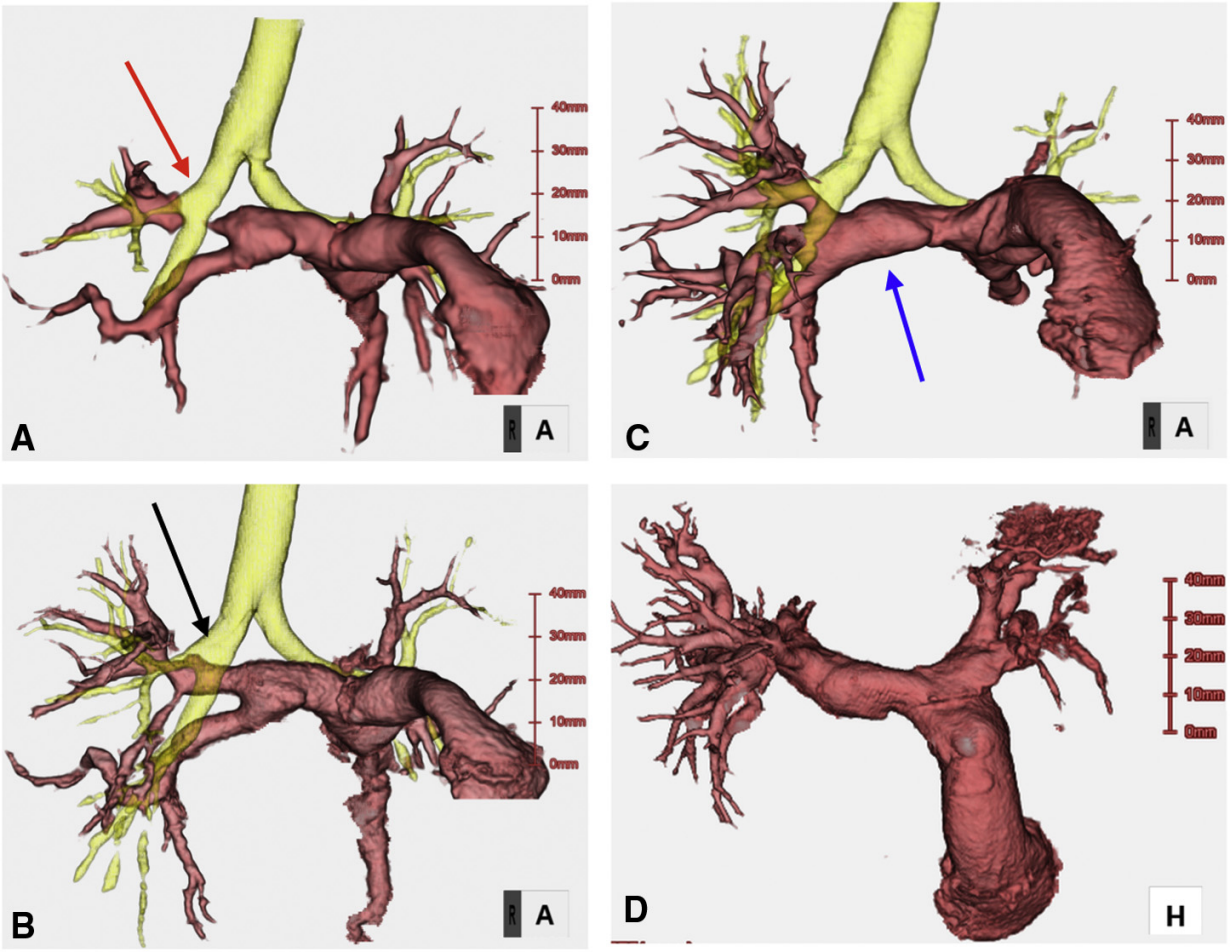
Pre- and postoperative 3-dimensional cardiac computed tomography scan of patient 4. A, Image taken after the first pulmonary artery augmentation with autologous pericardium. The distal right pulmonary artery is compressed by the right bronchus (*red arrow*). B, Image taken after pulmonary artery augmentation with tissue-engineered vascular graft patch for the first time. This patch was implanted at the stenotic region of the pulmonary artery (*black arrow*). C and D, Images taken after the final Rastelli operation. In the Rastelli operation, the graft was used again for the pulmonary artery (*blue arrow*).
